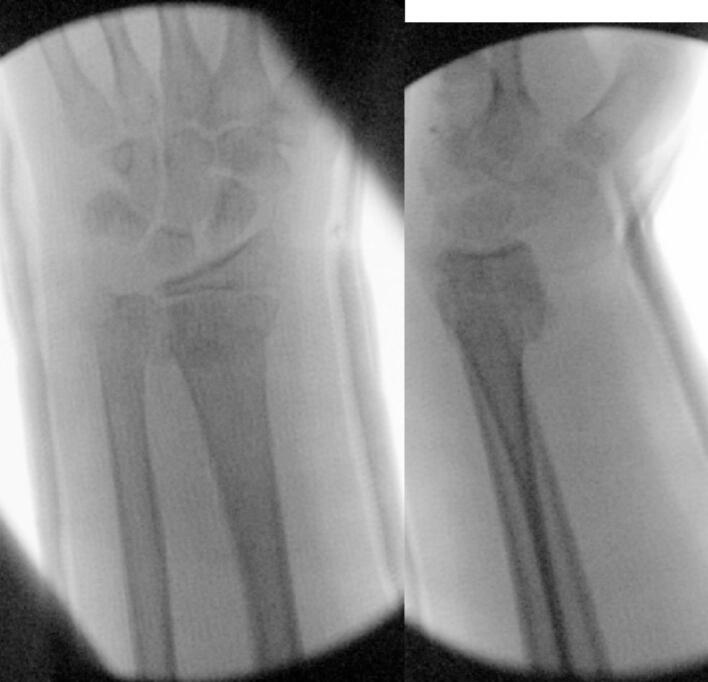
AP and lateral radiograph from intra-operative screening during closed reduction & casting.
